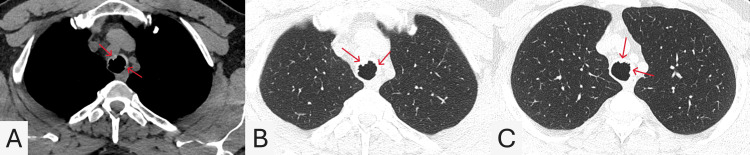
Axial non-contrast computed tomography (CT) chest images (A-C) (A) Soft tissue window shows irregular nodular thickening with calcified submucosal densities along the anterior tracheal wall (red arrows), sparing the posterior membranous wall. (B) Lung window highlights the extent of anterior wall nodularity (red arrows) without significant luminal narrowing. (C) A slightly lower lung window level shows submucosal densities (red arrows) with sparing of the posterior tracheal wall and a preserved airway lumen.
